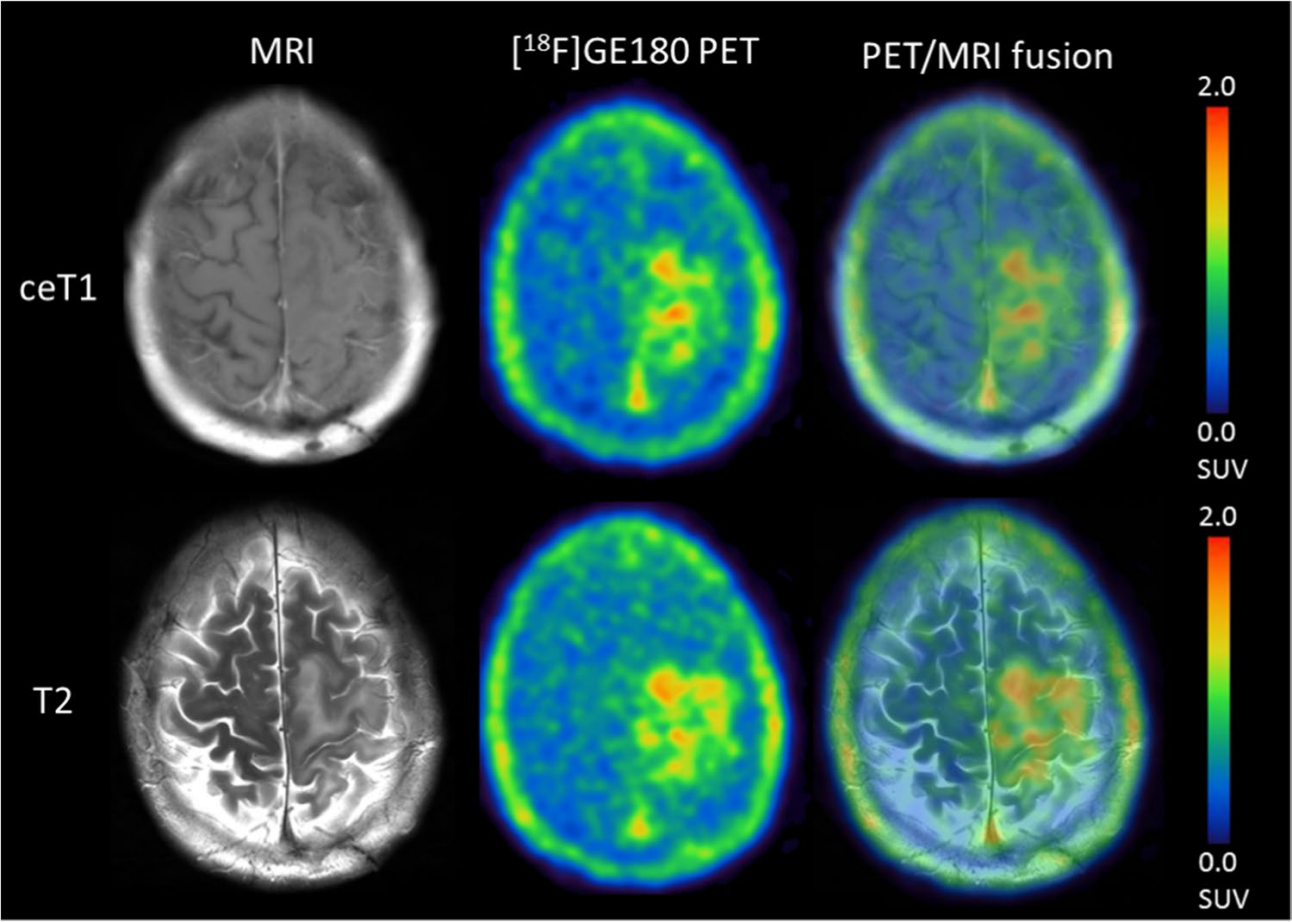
Exemplary case of a 39.5-year-old patient with recurrent astrocytoma, *IDH*-mutant, CNS WHO 2021 grade 3 (SUV_max_ 2.85). The patient progressed soon after re-radiochemotherapy and died 6 months after recurrence
